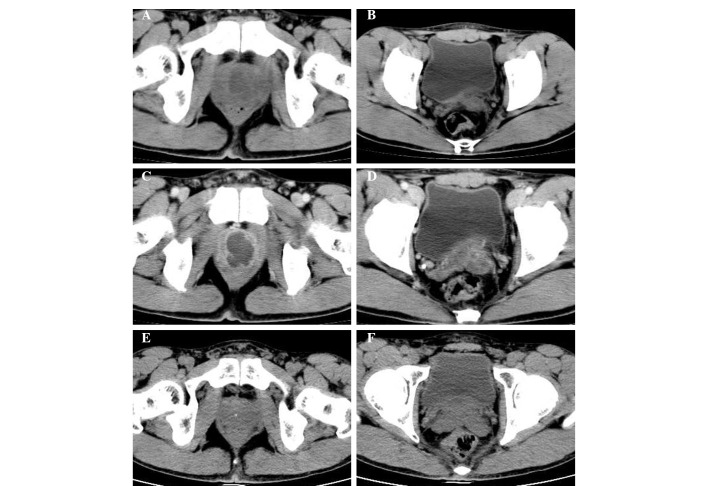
Pelvic computed tomography (CT) prior to and following prostate needle aspiration. (A and B) Noncontrast CT revealed multiple prostate abscesses, invading left seminal vesicle; (C and D) parenchymal phase CT images; (E and F) follow-up CT scans revealed a marked improvement in the prostate 1 month after the initial prostate needle aspiration.
